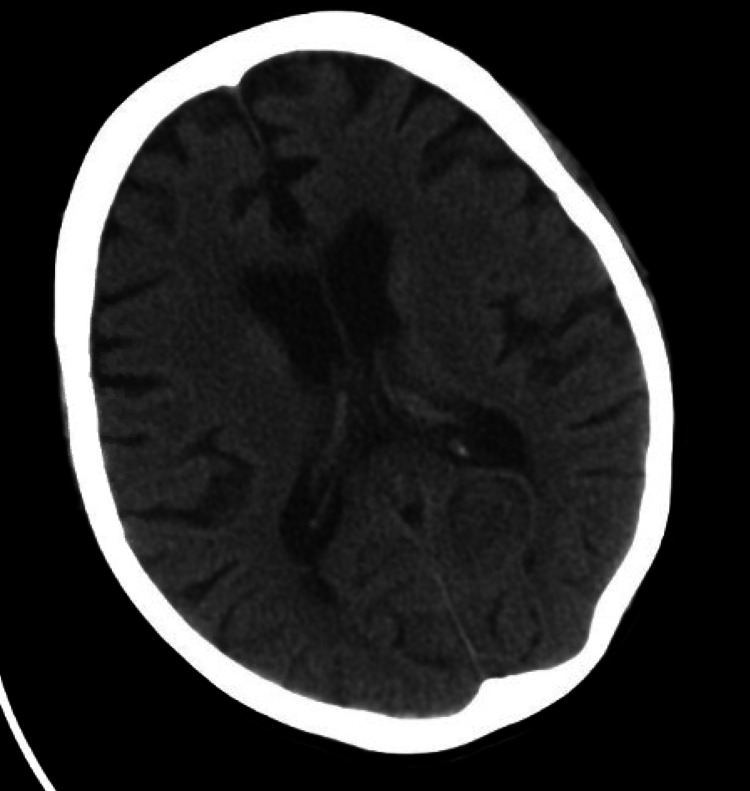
CT scan of the head: No acute intracranial abnormality
